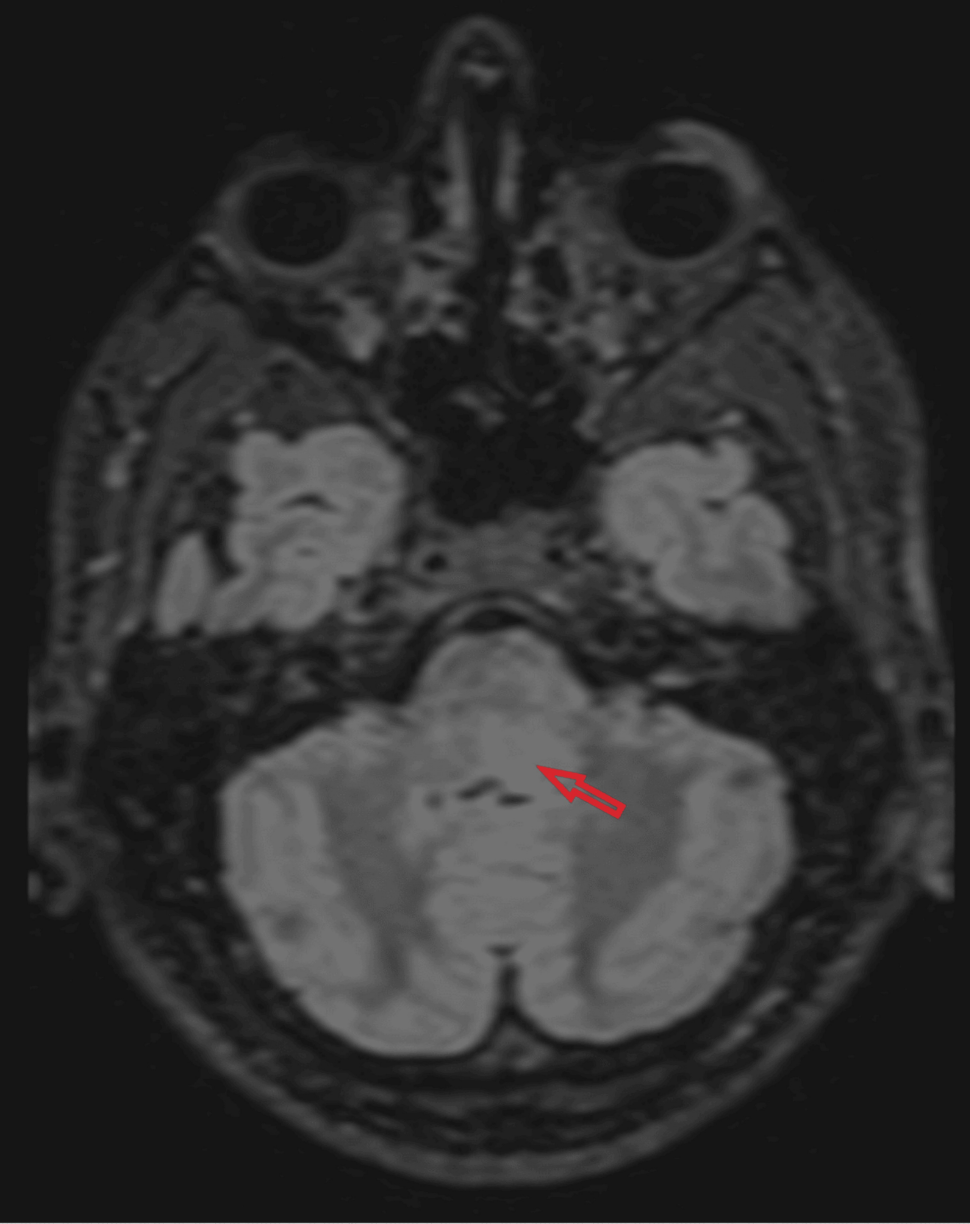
Axial fluid-attenuated inversion recovery (FLAIR) MRI demonstrating signal hyperintensity and edema predominantly in the left dorsal lower pons.
